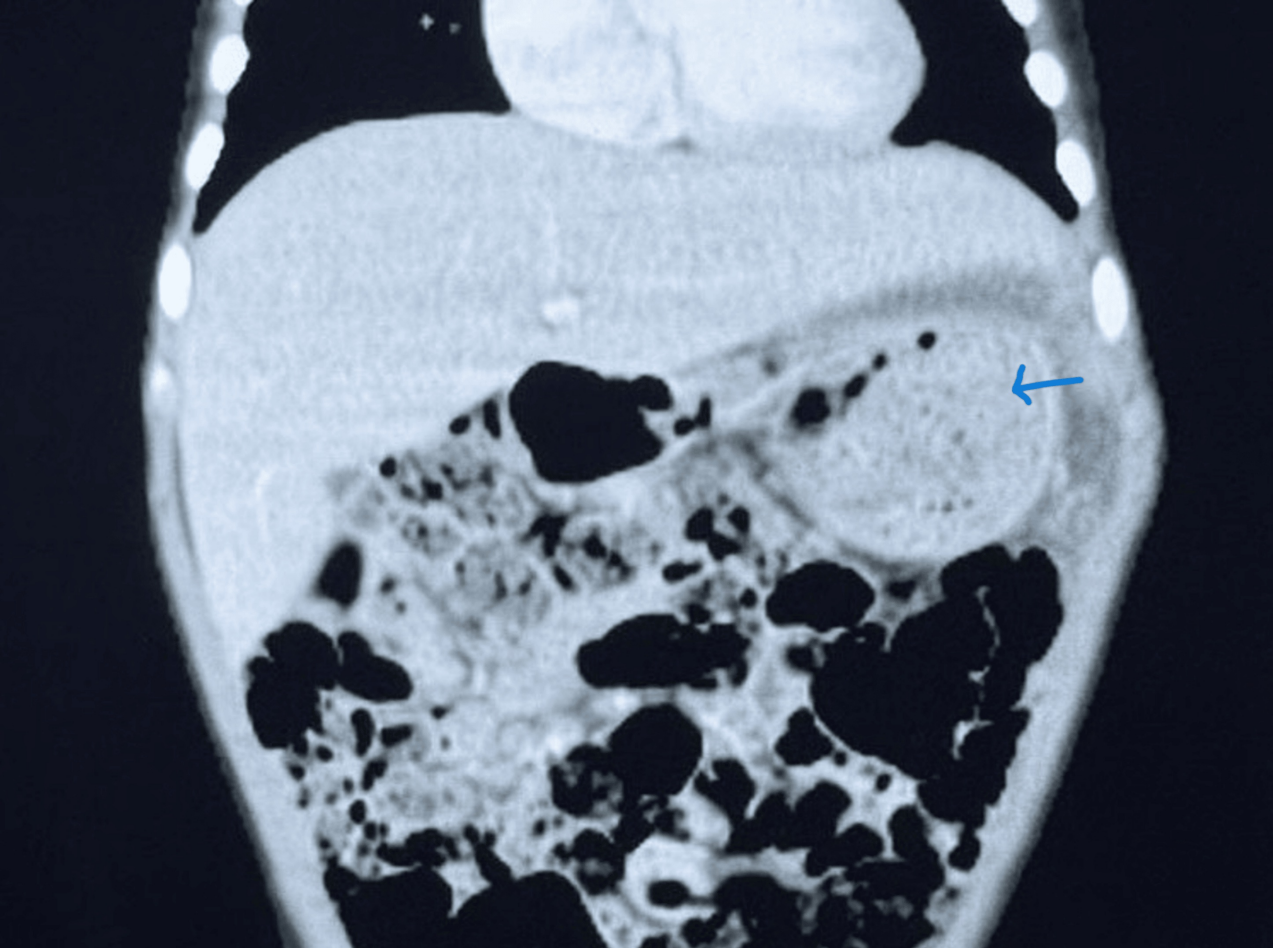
Coronal contrast-enhanced computed tomography (CT) of the abdomen Computed tomography (CT) of abdomen showing a 13 × 12 × 7.6 cm well-defined multiloculated cystic mass (blue arrow) with enhancing solid nodule and septa, superiorly abutting the diaphragm, laterally abutting the abdominal wall, and medially displacing the bowel loops, pancreas, and stomach.
